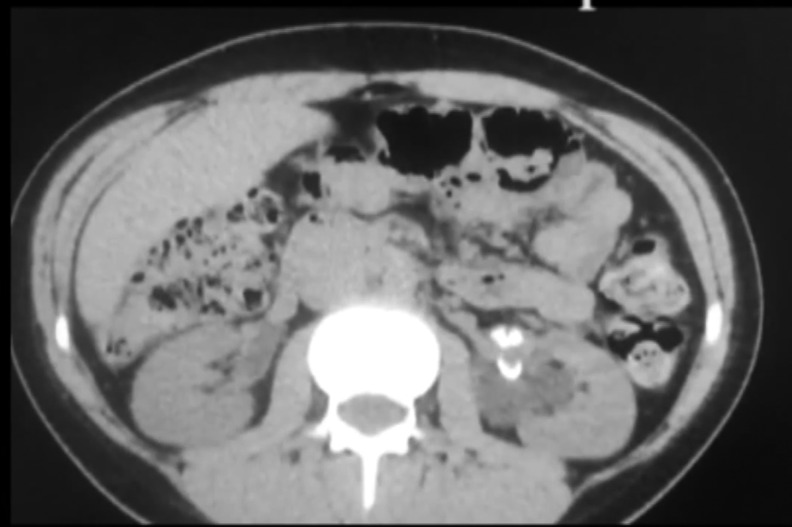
Noncontrast CT demonstrating nongravity-dependent calcifications at the *left* ureteropelvic junction with accompanying hydronephrosis.
